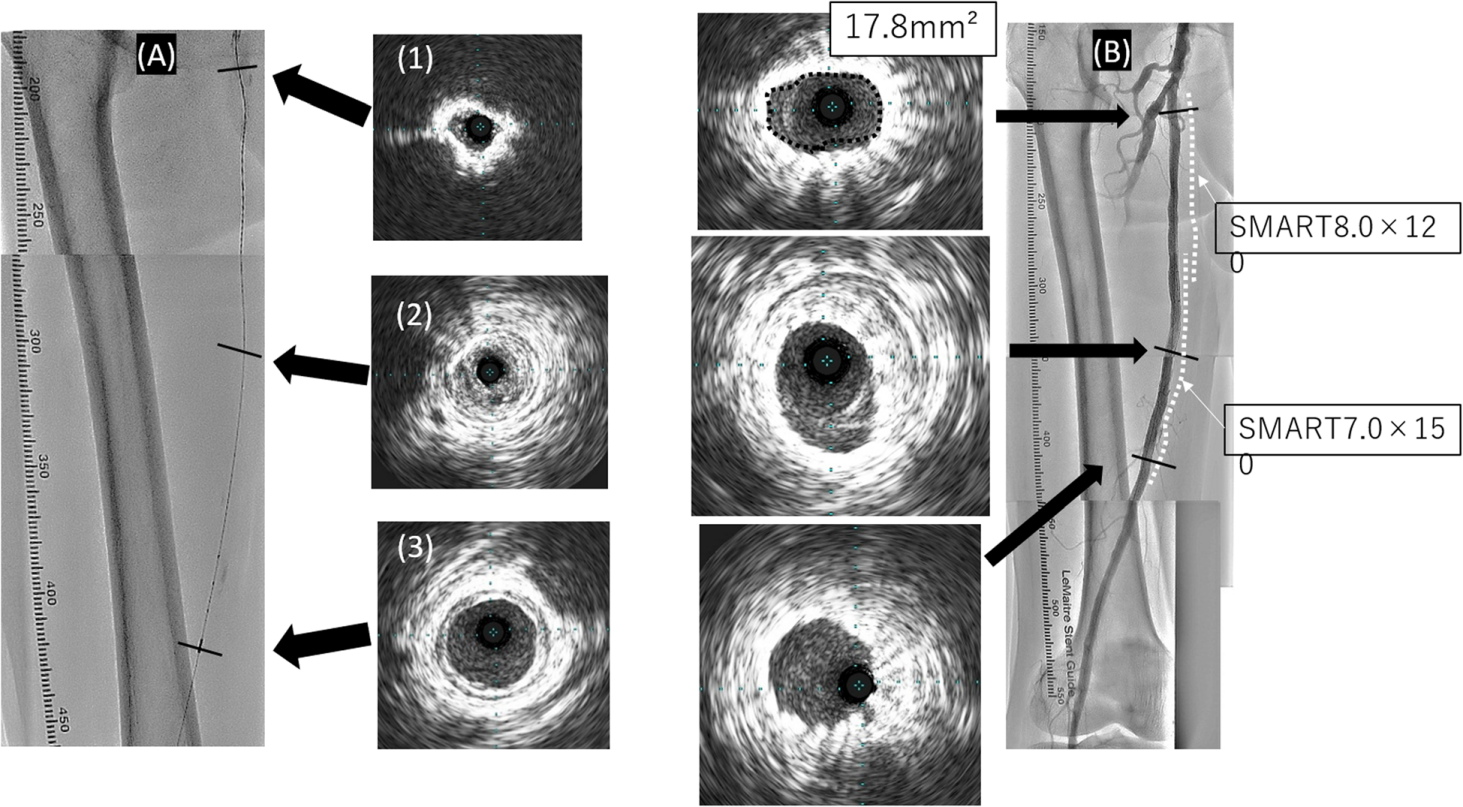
(A)IVUS images were obtained after the wire was passed through the CTO lesion. The vessel lumen at the ostium of the SFA was surrounded by calcification (1). The guidewire was passed through the center of the vessel (2). Distal reference of the CTO lesion (3). (B)Final angiography and IVUS. Acceptable expansion of the stent was achieved at the SFA ostium (17.8 mm²). Also, no plaque shift to the DFA was observed. IVUS, Intravascular ultrasound; CTO, chronic total occlusion; SFA, superficial femoral artery; DFA, deep femoral artery
